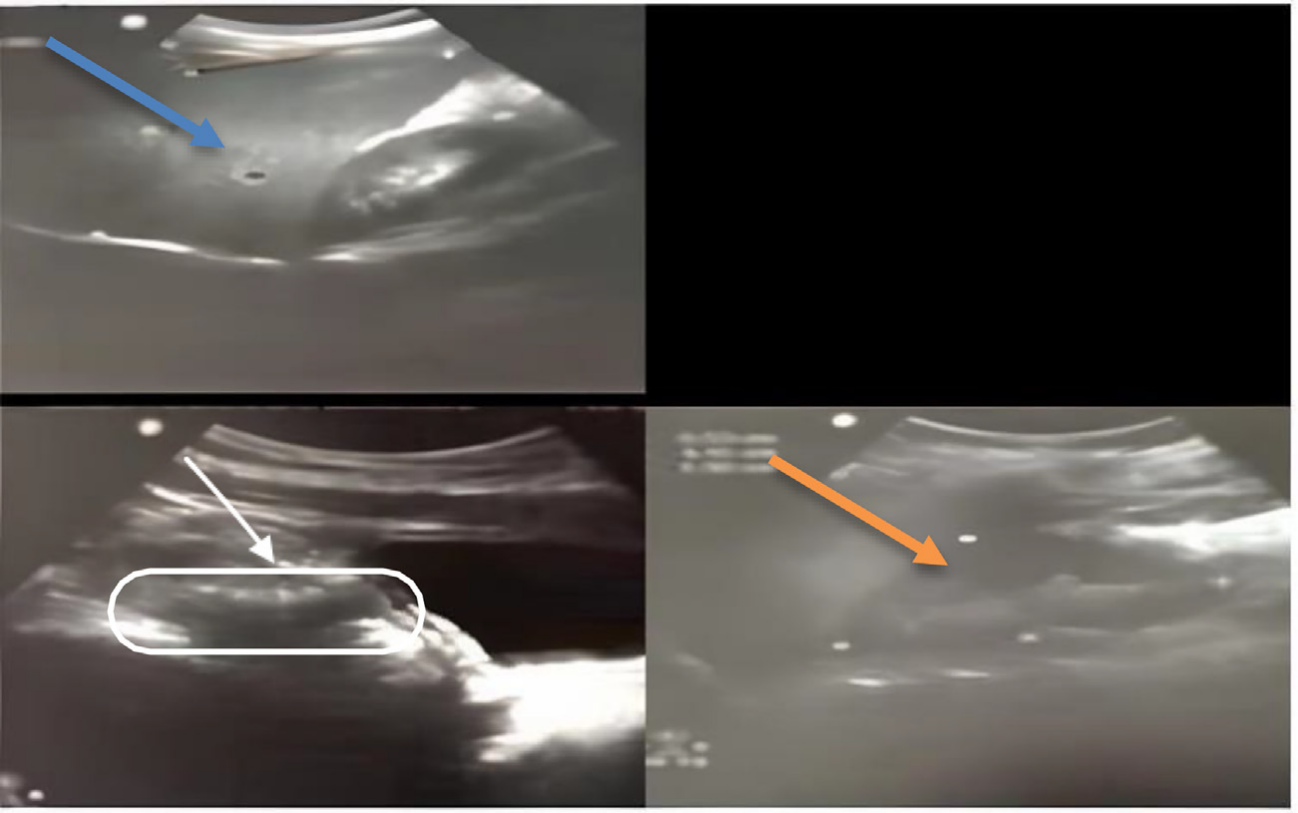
Pelviabdominal ultrasonography showing an peristaltic bowel loop in the right iliac fossa with distended distal part reaching about 7.5 mm and collapsed proximal half with echogenic wall till its origin and its origin seen inferior to the right of the umbilicus. A Minimal rim of fluid is seen around the peristaltic bowel loop and colonic gas distention is apparent.
